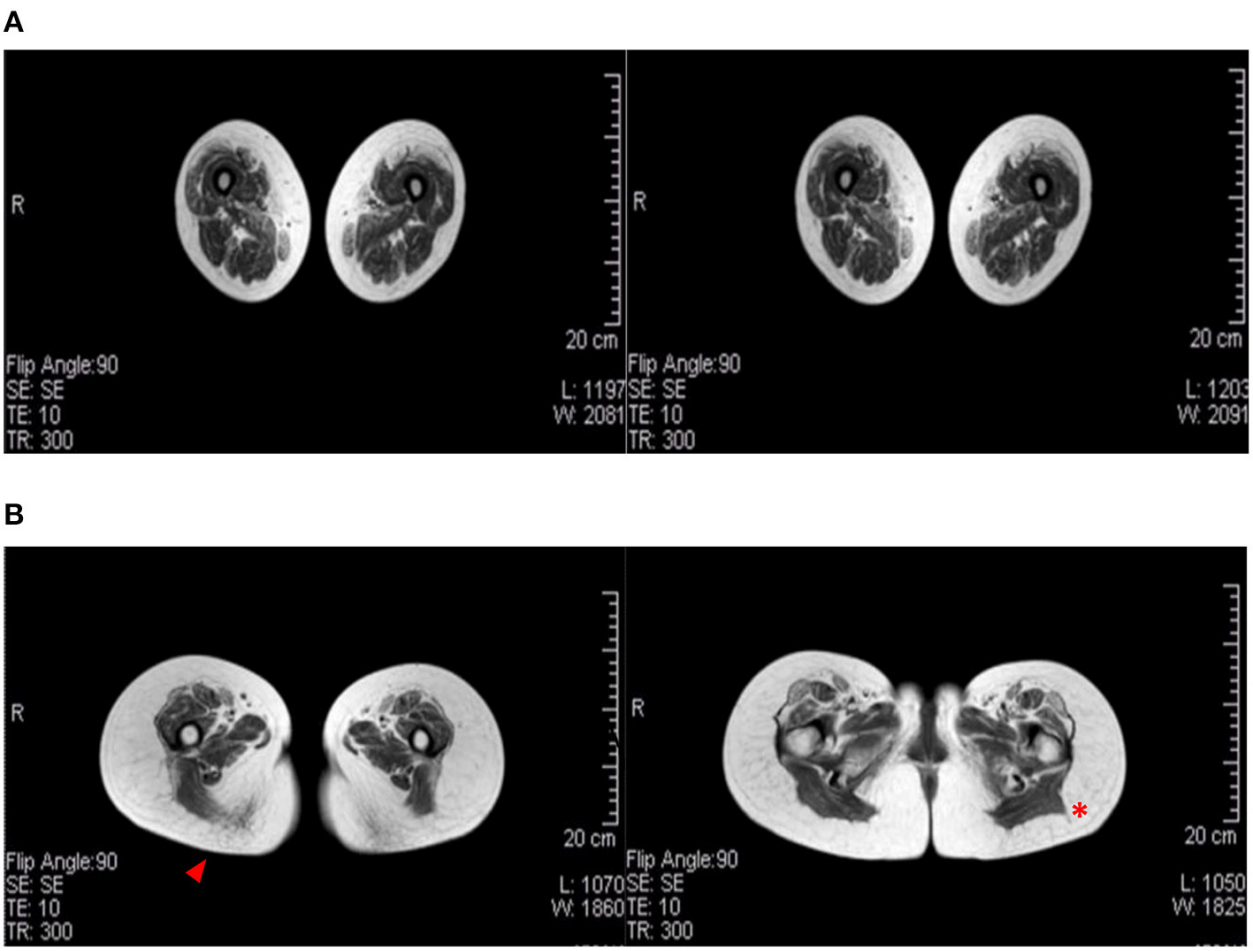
Muscular MRI radiological aspects. (A) A female patient (age 60) affected by LGMD D2 and mother of (B) the index female case of the Italian branch of the Italo-Spanish family (age 34). Advanced replacement and atrophy of the semitendinous (arrowhead) compared to the back muscles of the thigh of the wide side and the femoral rectus of the middle and small gluteus (red asterisk) to the illicit insertion.
